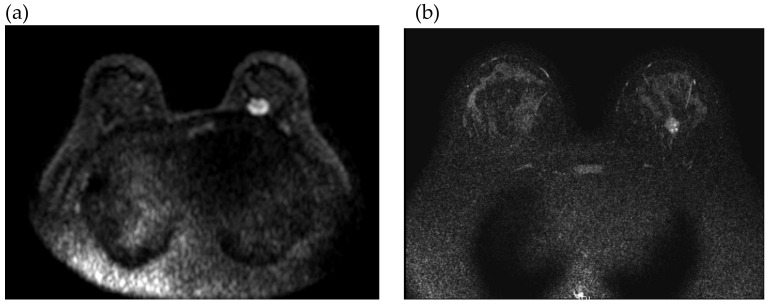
Imaging of the breasts on magnetic resonance imaging (MRI). (a) Breast MRI of patient No. 6 at the first diagnosis of breast cancer in October 2013. (b) Breast MRI of patient No. 6’s sister at the first diagnosis of breast cancer in January 2016.
